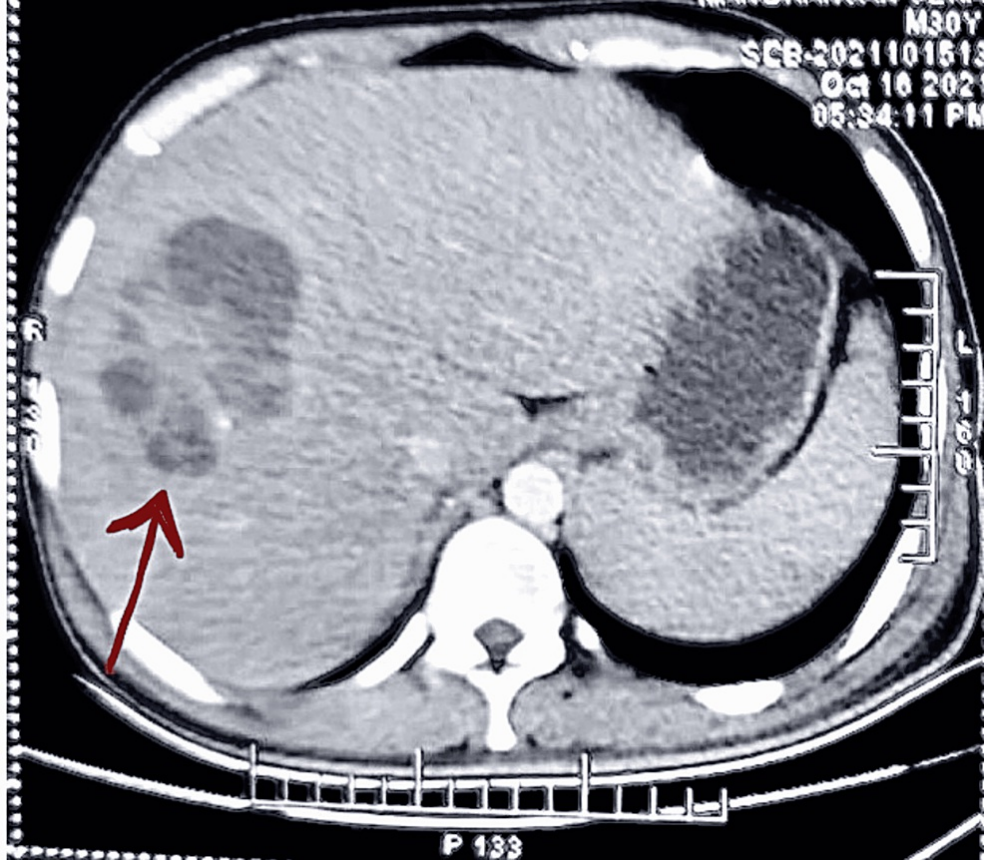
Contrast-enhanced computed tomography of the abdomen of Case 1 showing honeycomb pattern liver abscess (arrow)
